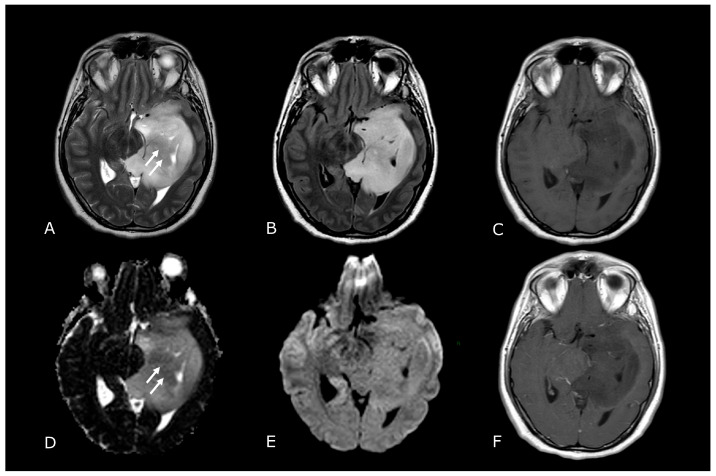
Morphological sequences: tumor cellularity. Morphological sequences: tumor cellularity. Left deep temporo-mesial WHO grade 3 IDH-mut diffuse astrocytoma. Lesion is hyperintense on T2w and FLAIR images (A,B), isointense on DWI (E), with areas of lower T2 signal intensity and diffusion restriction (white arrows in (A,D) that reflect hypercellular and probably more anaplastic tissue. No contrast enhancement is detectable on post-contrast T1w image (F) compared to pre-contrast image (C).
